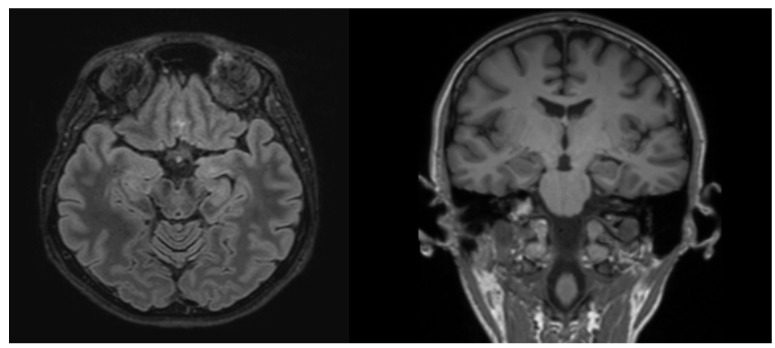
Brain MRI (3T) axial T2-FLAIR (left) showing a small gliosis area (~2.5 mm) in the left amygdala and coronal T1 (right) slight hippocampal asymmetry due to incomplete left hippocampal inversion.
